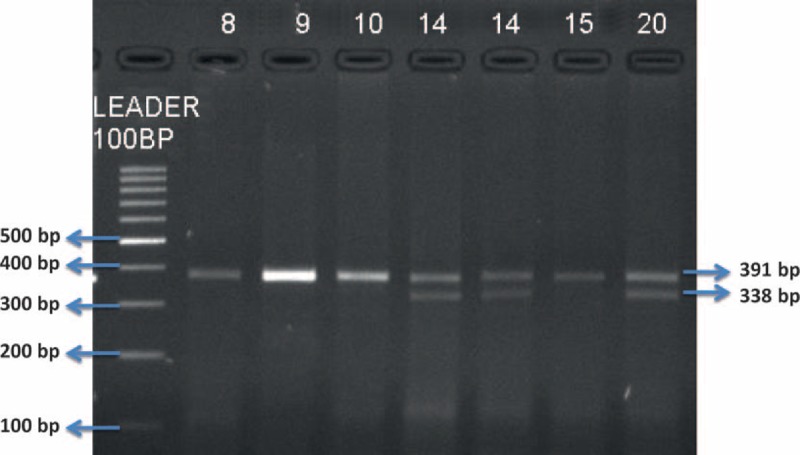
MMP2 −735 CT polymorphism agarose gel electrophoresis image after RFLP. MMP = matrix metalloproteinase, RFLP = restriction fragment length polymorphism.
